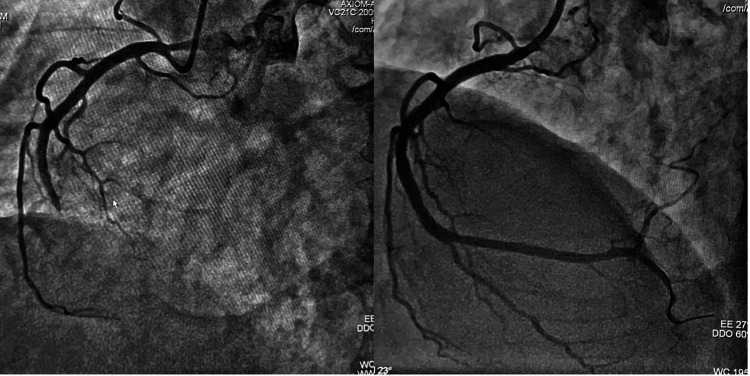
Coronary angiography Coronary angiography demonstrating the complete thrombotic occlusion of the proximal-to-mid RCA (TIMI 0 flow) and successful stenting with the restoration of TIMI 3 flow. These findings confirmed isolated right ventricular involvement despite preserved left ventricular function. Echocardiographic frames were unavailable; findings are described narratively in the text. The left coronary system was angiographically normal (image not included for brevity). RCA: right coronary artery; TIMI: Thrombolysis in Myocardial Infarction
